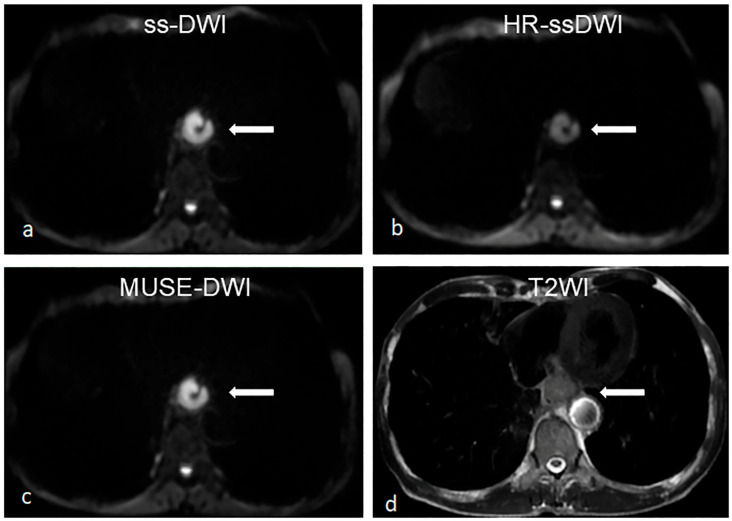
Images acquired from a 56-year-old male esophageal cancer patient with ss-DWI (a), HR-ssDWI (b), MUSE-DWI (c), and T2-weighted (d) sequences. There is no significant difference in image quality, esophageal contour and lesion conspicuity between MUSE-DWI and ss-DWI. However, compared to HR-ssDWI, both have better lesion conspicuity and lower image distortion. The arrow indicates the esophageal tumor.
